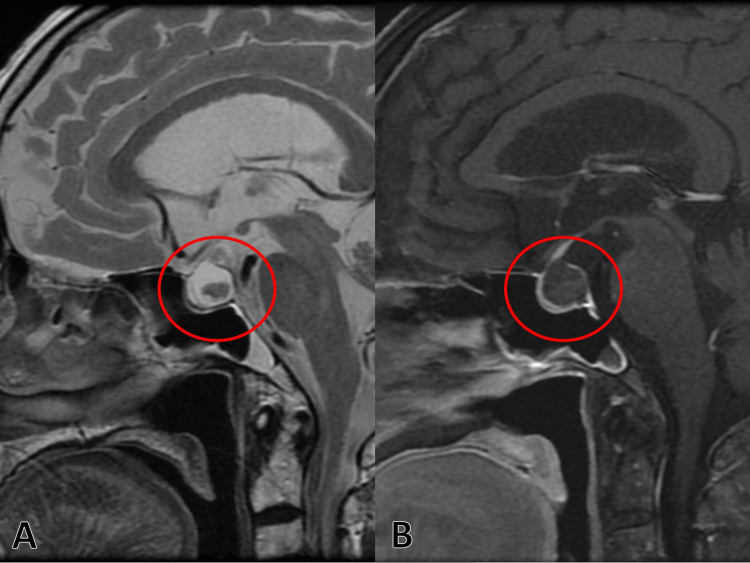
Brain contrast-enhanced magnetic resonance imaging (MRI) with T2-weighted imaging showing high signal intensity with internal low-signal areas (A) and no contrast enhancement (B), consistent with a Rathke’s cleft cyst (red circles)
